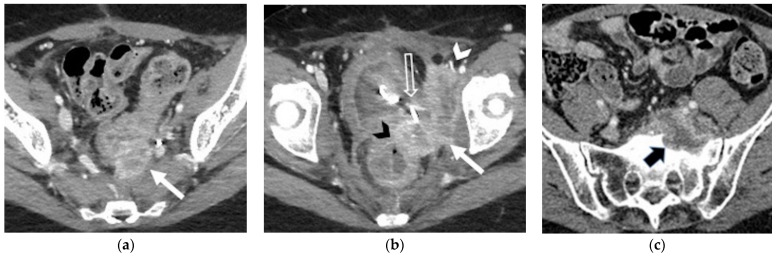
(a,b) A 36-year-old patient who underwent radical hysterectomy with bilateral salpingo–oophorectomy (BSO) and chemoradiation therapy for cervical cancer. Axial post-contrast CT images show pathological tissue infiltrating the left piriform muscle, the left internal obturator muscle (solid white arrow in (a,b)) and the external iliac vein (white arrowhead in (b)). Note the infiltration of the anterior rectal wall (black arrowhead in (b)), the bladder and the left ureteral orifice (with the ureteral stent) (open arrow in (b)). (c) A 58-year-old who underwent radical hysterectomy with BSO for cervical cancer. Axial post-contrast CT image shows pathological tissue infiltrating the left sacral promontory (black arrow).
